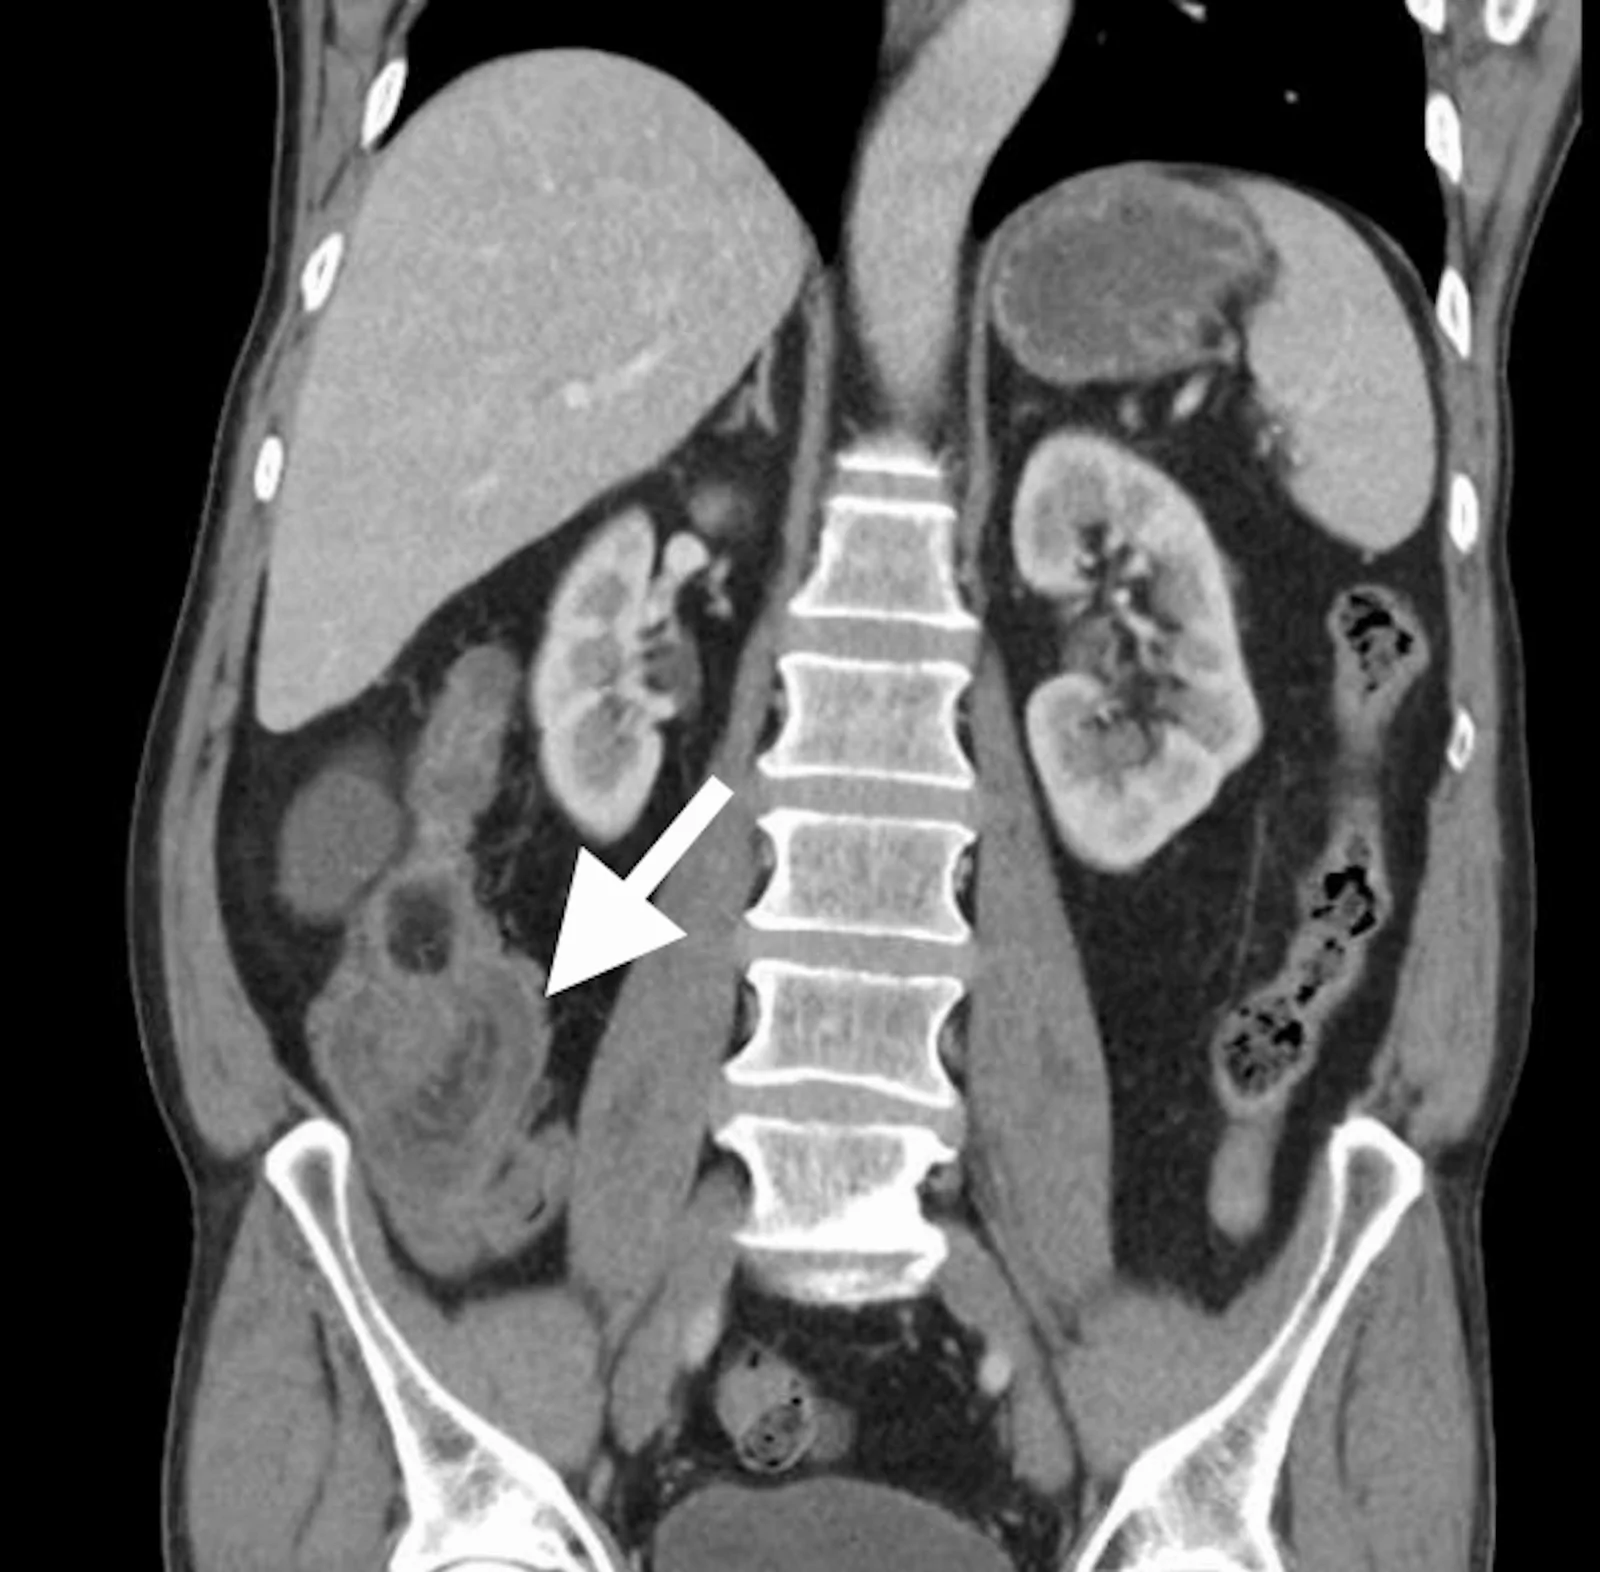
Coronal view of the CT abdomen Pathognomonic telescoping ("target sign") is visualized in the patient's right lower quadrant, as indicated by the arrow. CT, computed tomography
